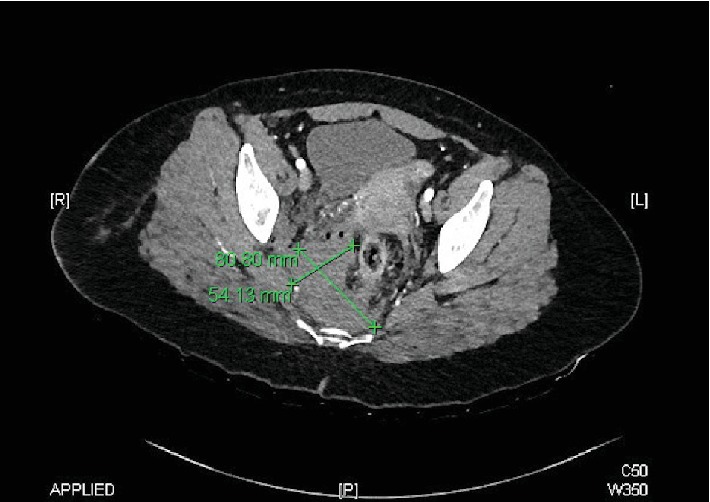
Presacral hematoma in the pelvic CT.
